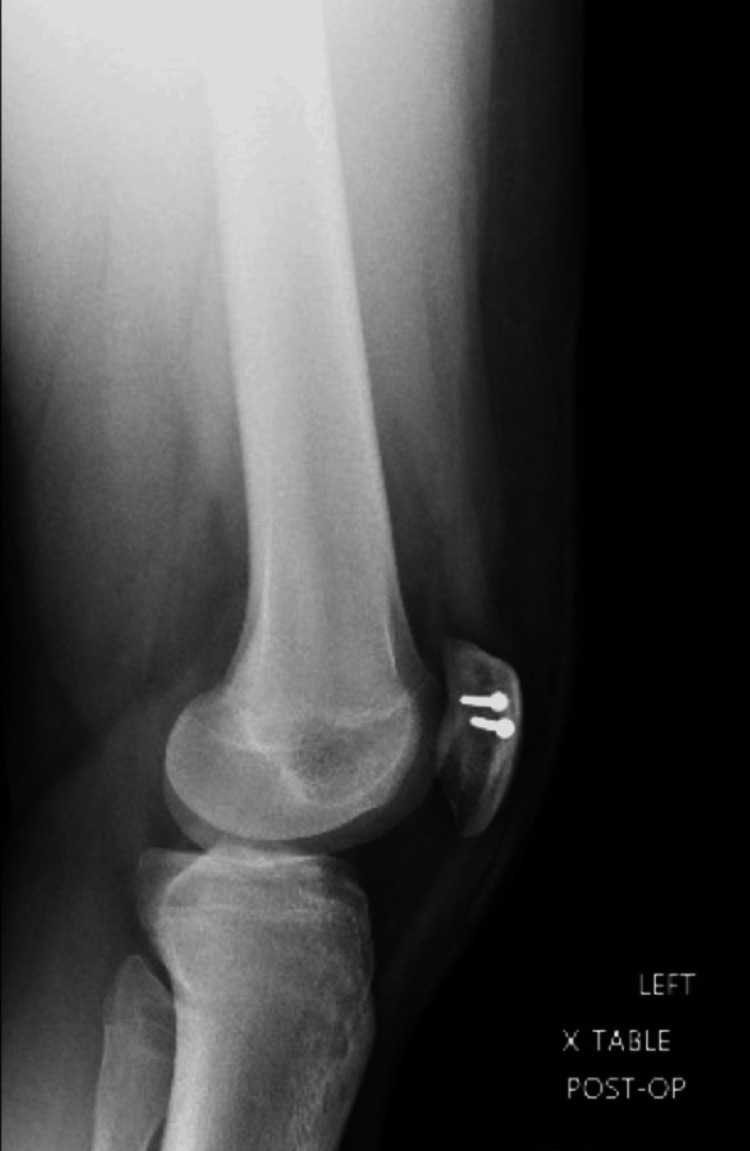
Postoperative X-ray L knee lateral view
